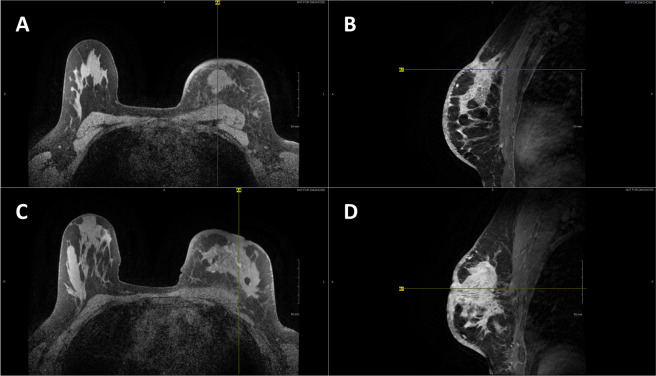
Axial (A) and sagittal (B) T1 contrast-enhanced MR images of Subject 03’s superior hotspot. Axial (C) and sagittal (D) T1 contrast-enhanced MR images of Subject 03’s nipple hotspot. Localized regions of skin retraction and skin thickening evident from MR images.
